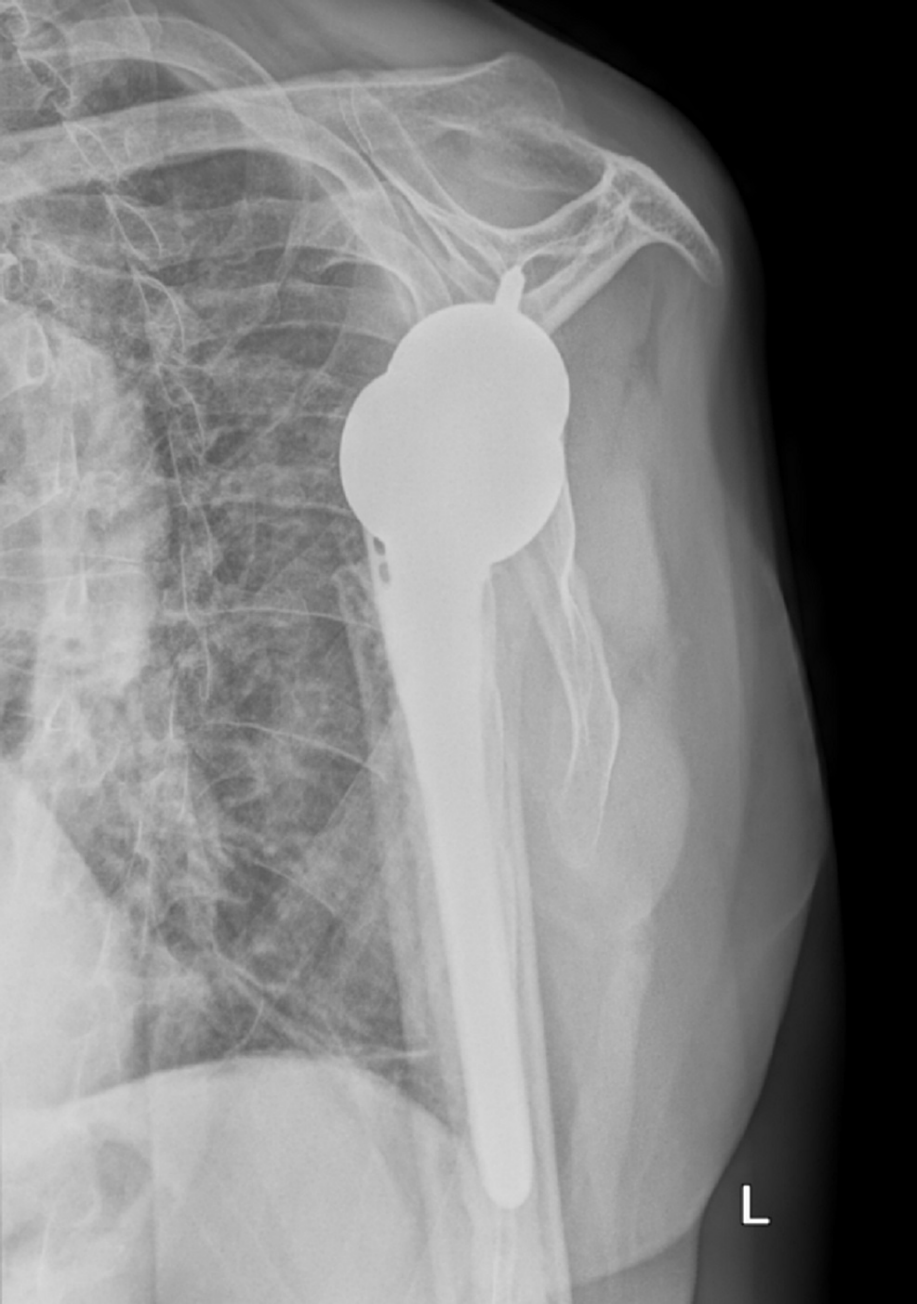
Scapular Y postoperative radiograph of the left shoulder at 12 weeks demonstrates a reverse total shoulder arthroplasty (rTSA) in satisfactory alignment without signs of loosening or failure.
